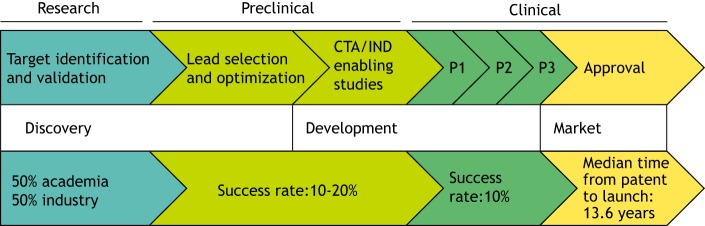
Pathway for drug development from discovery to marketing approval. Around 50% of new drugs were first reported by academic publications (Patridge et al., 2015). Following target identification and validation, translational scientists perform hit-finding/lead clinical candidate optimization and identification. Once the lead clinical candidate is identified, preclinical development studies are then performed to prepare for filing the appropriate applications with regulatory agencies, such as the CTA (Clinical Trial Application) in the EU or the IND (Investigational New Drug) in the USA. For neuromuscular disease, 43% of therapies in development are small molecules, whilst 23% are combined gene and antisense oligonucleotide (ASO) technologies. Nearly 50% of all neuromuscular disease therapies in development are focused on spinal muscular atrophy (SMA) and Duchenne's muscular dystrophy (DMD). If permitted, drugs may then be tested in phase 1/2/3 (P1/P2/P3) clinical trials. The median time from first patent (discovery) to launch (FDA approval) of all approved drugs was 13.6 years in 2018. Statistics from Aitken et al. (2018) and Patridge et al. (2015).
